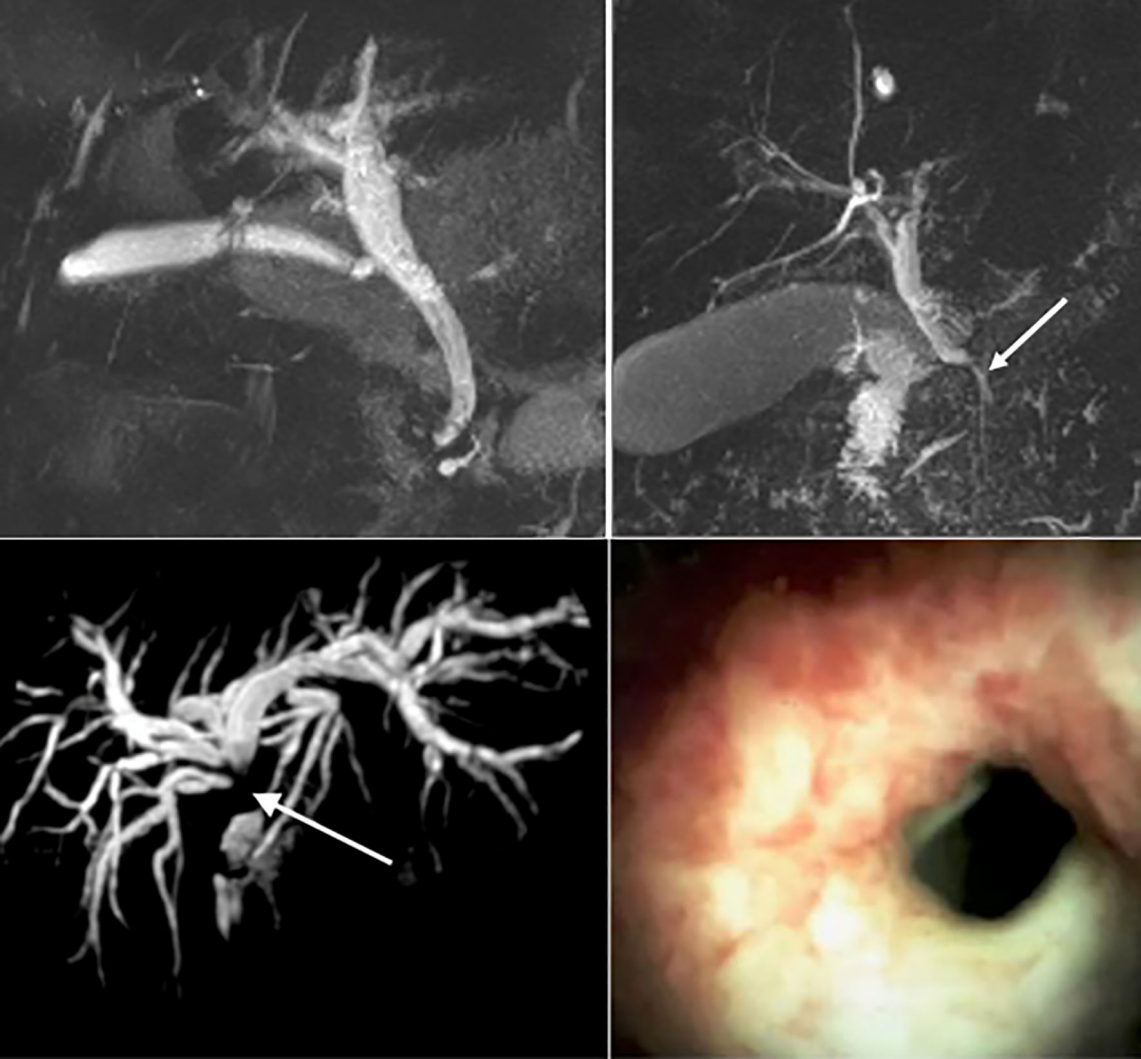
Top left: Magnetic resonance imaging (MRI) of a normal biliary tree. Top right: MRI imaging of a benign biliary stricture secondary to acute pancreatitis (white arrow). Bottom Left: MRI of a dilated intrahepatic biliary tree secondary to a cholangiocarcinoma at the liver hilum (white arrow). Bottom right: Cholangioscopy demonstrating mucosal nodularity and neovascularisation in the 7 -o’clock position, secondary to cholangiocarcinoma.
